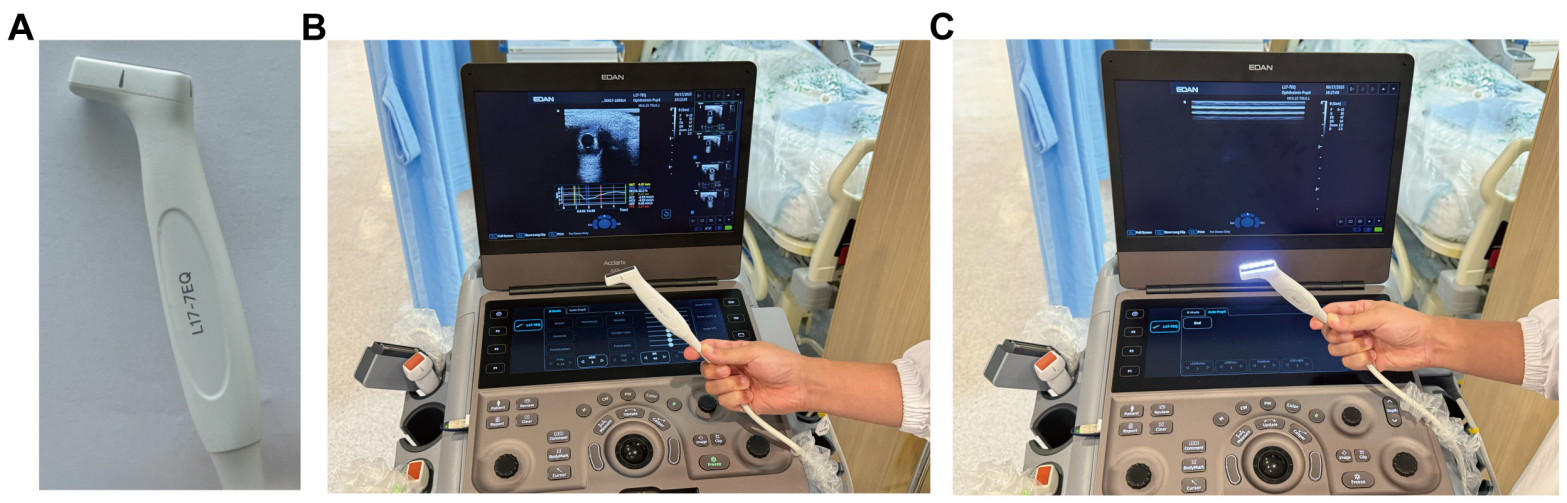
Automated ultrasound pupillometry system. (A) Handheld linear ocular probe (L17–7EQ) used for transpalpebral imaging. (B) EDAN AX8 ultrasound system in the “Ophthalmic-Pupil” preset, displaying the B-mode ocular image. (C) Auto-UPA pupillometry mode with the integrated light source activated, ready for automated pupillary light reflex acquisition.
